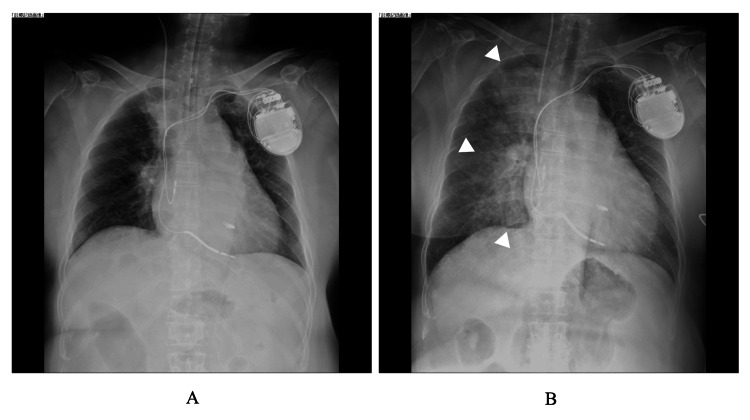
Chest radiograph at the end of transcatheter edge-to-edge repair with MitraClip® procedure (A), at the onset of hypertension and shivering (B). The butterfly shadow (△) is more pronounced at the symptom onset than at the end of the procedure.
